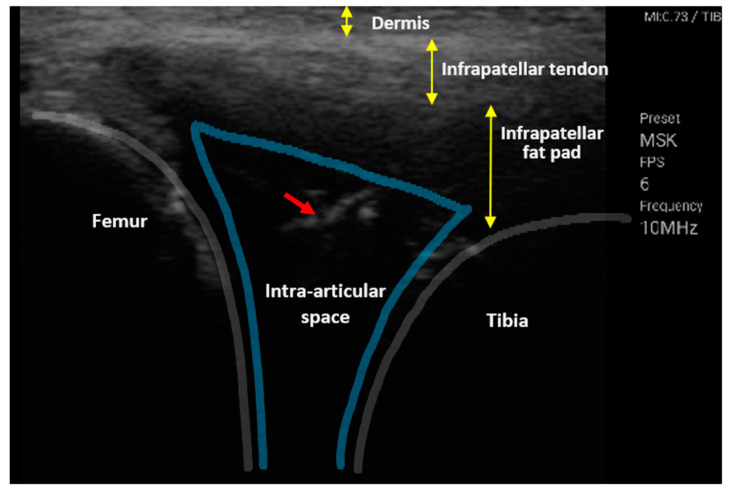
Ultrasonography image that was captured when the needle electrode entered the intra-articular space. The red small arrow shows the needle location and the different depths of the tissue layers indicated by the yellow arrows. The blue boundary shows intra-articular space.
